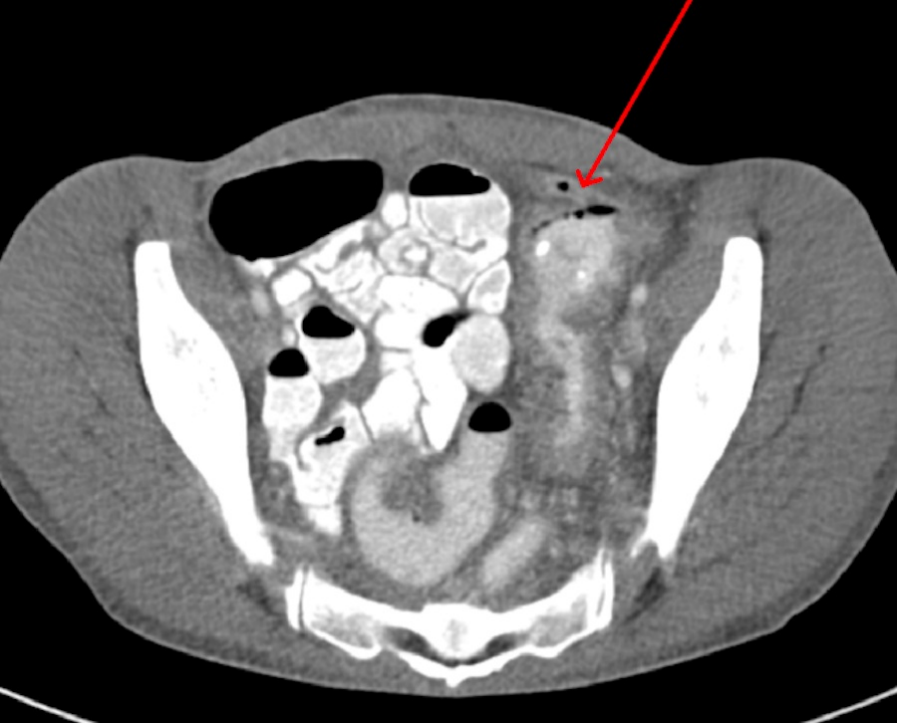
Computed tomographic (CT) scan of the abdomen and pelvis showing small tract (red arrow) extending from the sigmoid colon towards the bladder dome suggestive of fistulous communication
